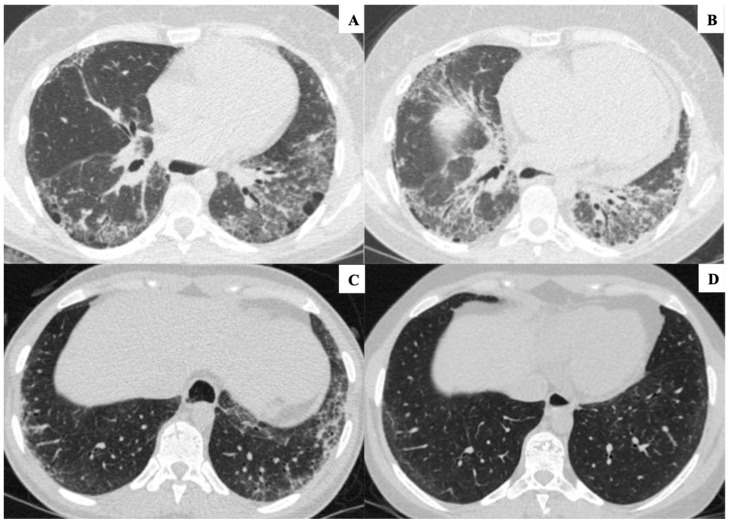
(A,B): axial HRCT images of a patient at different follow-up times (in (A), baseline scan and, in (B), FU2 near to 60 months), demonstrating radiological progression of ILD, characterized by increased extent of interstitial opacities and volume loss (in this case, notably in the lower lobes). (C,D): axial HRCT images of another patient at different follow-up times (in (C), baseline scan, and in (D), FU2), demonstrating radiological improvement of ILD, characterized by a reduction in interstitial opacities in the lower lung zones, particularly on the left.
